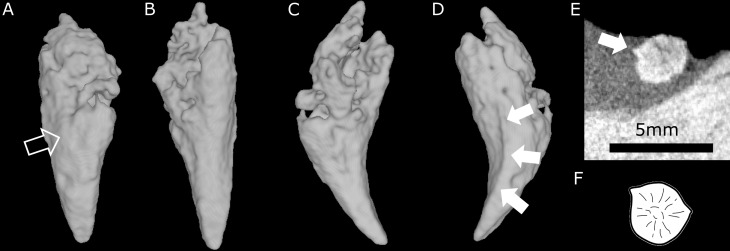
Three-dimensional rendering of the ex situ incisor of BP/1/4009. A, labial view (the empty arrow points to the wear facet); B, lingual view; C, mesial view; D, distal view; E, cross CT section through the middle of the ex situ incisor showing the conspicuous ridge (white arrow); F, cross section of the first right incisor of the lower jaw of an unidentified scylacosaurid therocephalian (= *Glanosuchus*?), redrawn after [51, 52].
